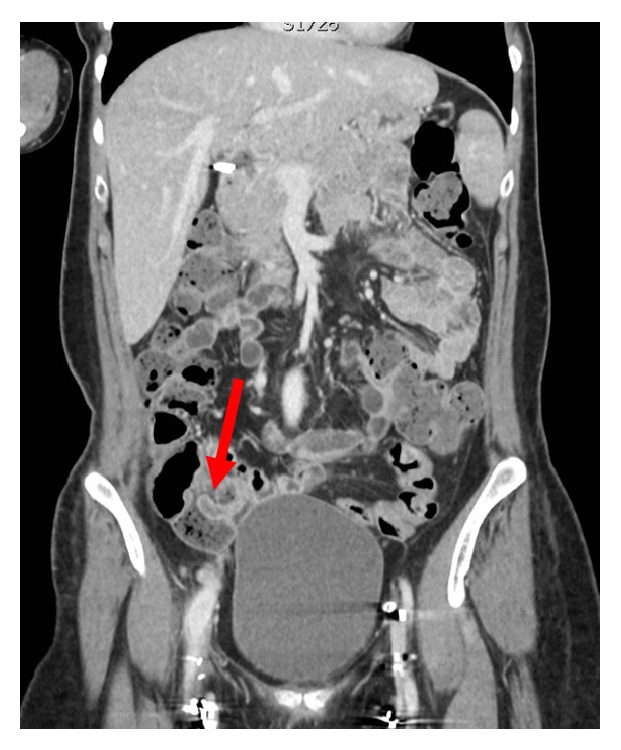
Coronal contrast enhanced CT image demonstrates a long, hypodense tubular structure representing a normal appendix (arrow) invaginating in the lumen of the cecal base.
